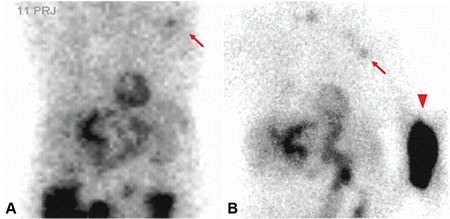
An 84-year-old female with a long history of asthma presented with an episode of chest pain and severe hypertension. The patient denied coronary angiography. Thus, a SPECT myocardial perfusion imaging (MPI) with dobutamine protocol was performed. Anterior projection of the raw cinematic image of stress SPECT MPI study (A) revealed a faint focal uptake in the region of left axilla (shown by arrow) as well as noticeably poor count statistics. In order to confirm the presence of tracer extravasation in the injection site, a planar anterior image with arms by the side (B) was obtained. As can be seen in B, considerable dose extravasation in the left forearm (shown by arrowhead) as well as a faintly hot axillary node ipsilateral to injection site were noted.
